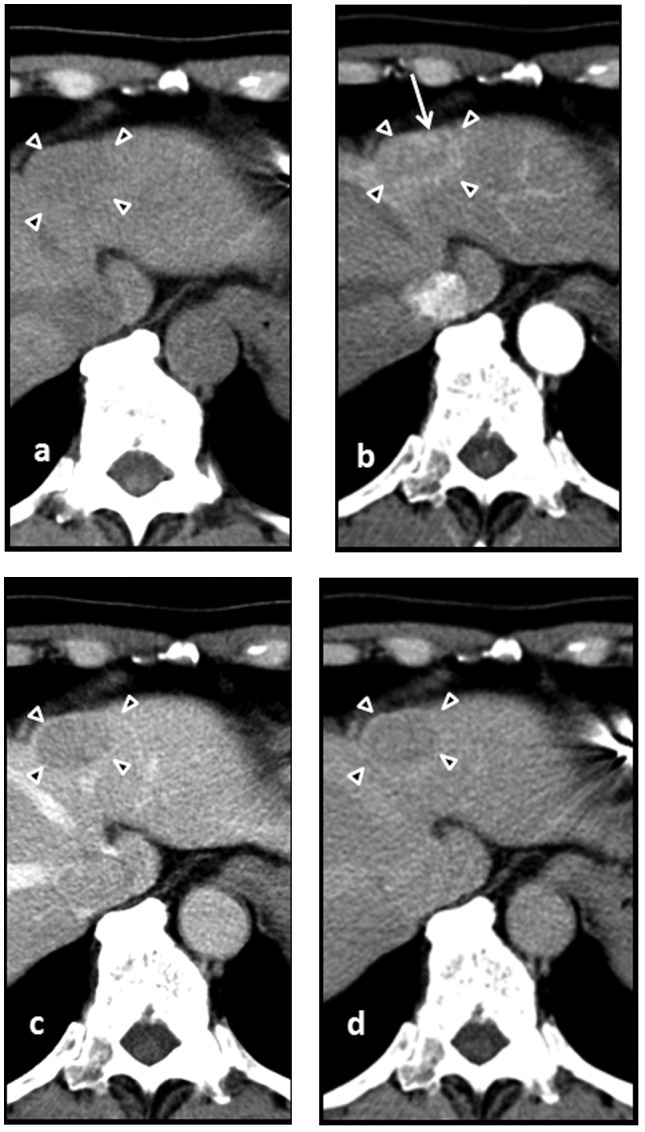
Contrast-enhanced computed tomography performed at the time of admission. A tumor 30 mm in size was observed in the S2 liver segment (arrowheads). A high density was indicated inside the tumor in the arterial phase (arrows), while a low density was indicated in the portal and delayed phases. (a) Plain; (b) arterial phase; (c) portal phase; (d) delayed phase.
